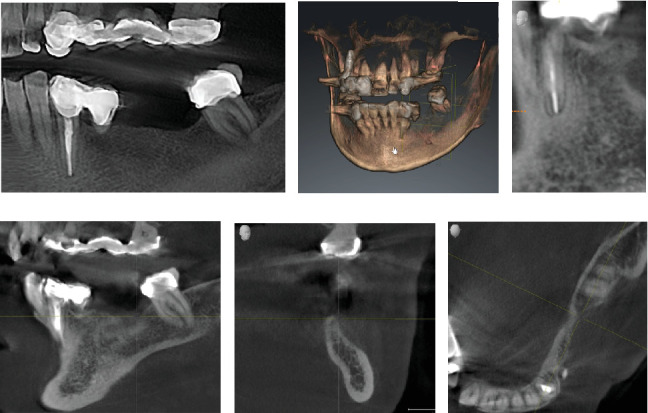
(a) Initial OPG showing a large defect between Sites 34 and 37, (b) CBCT of defect site, (c) CBCT image of the apical lesion in Site 33, and (d–f) CBCT perspectives of defect site demonstrating severe bone loss.
